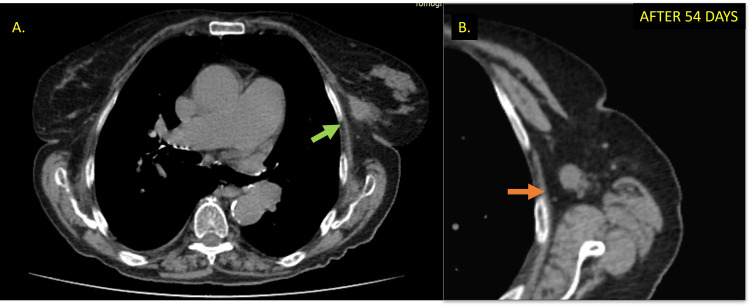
Chest computed tomography after the percutaneous biopsy. The mass described in the mammography (A) showed a reduction in dimension (green arrow). An enlargement and atypical lymph (B) node is observed in the axilla (orange arrow).
